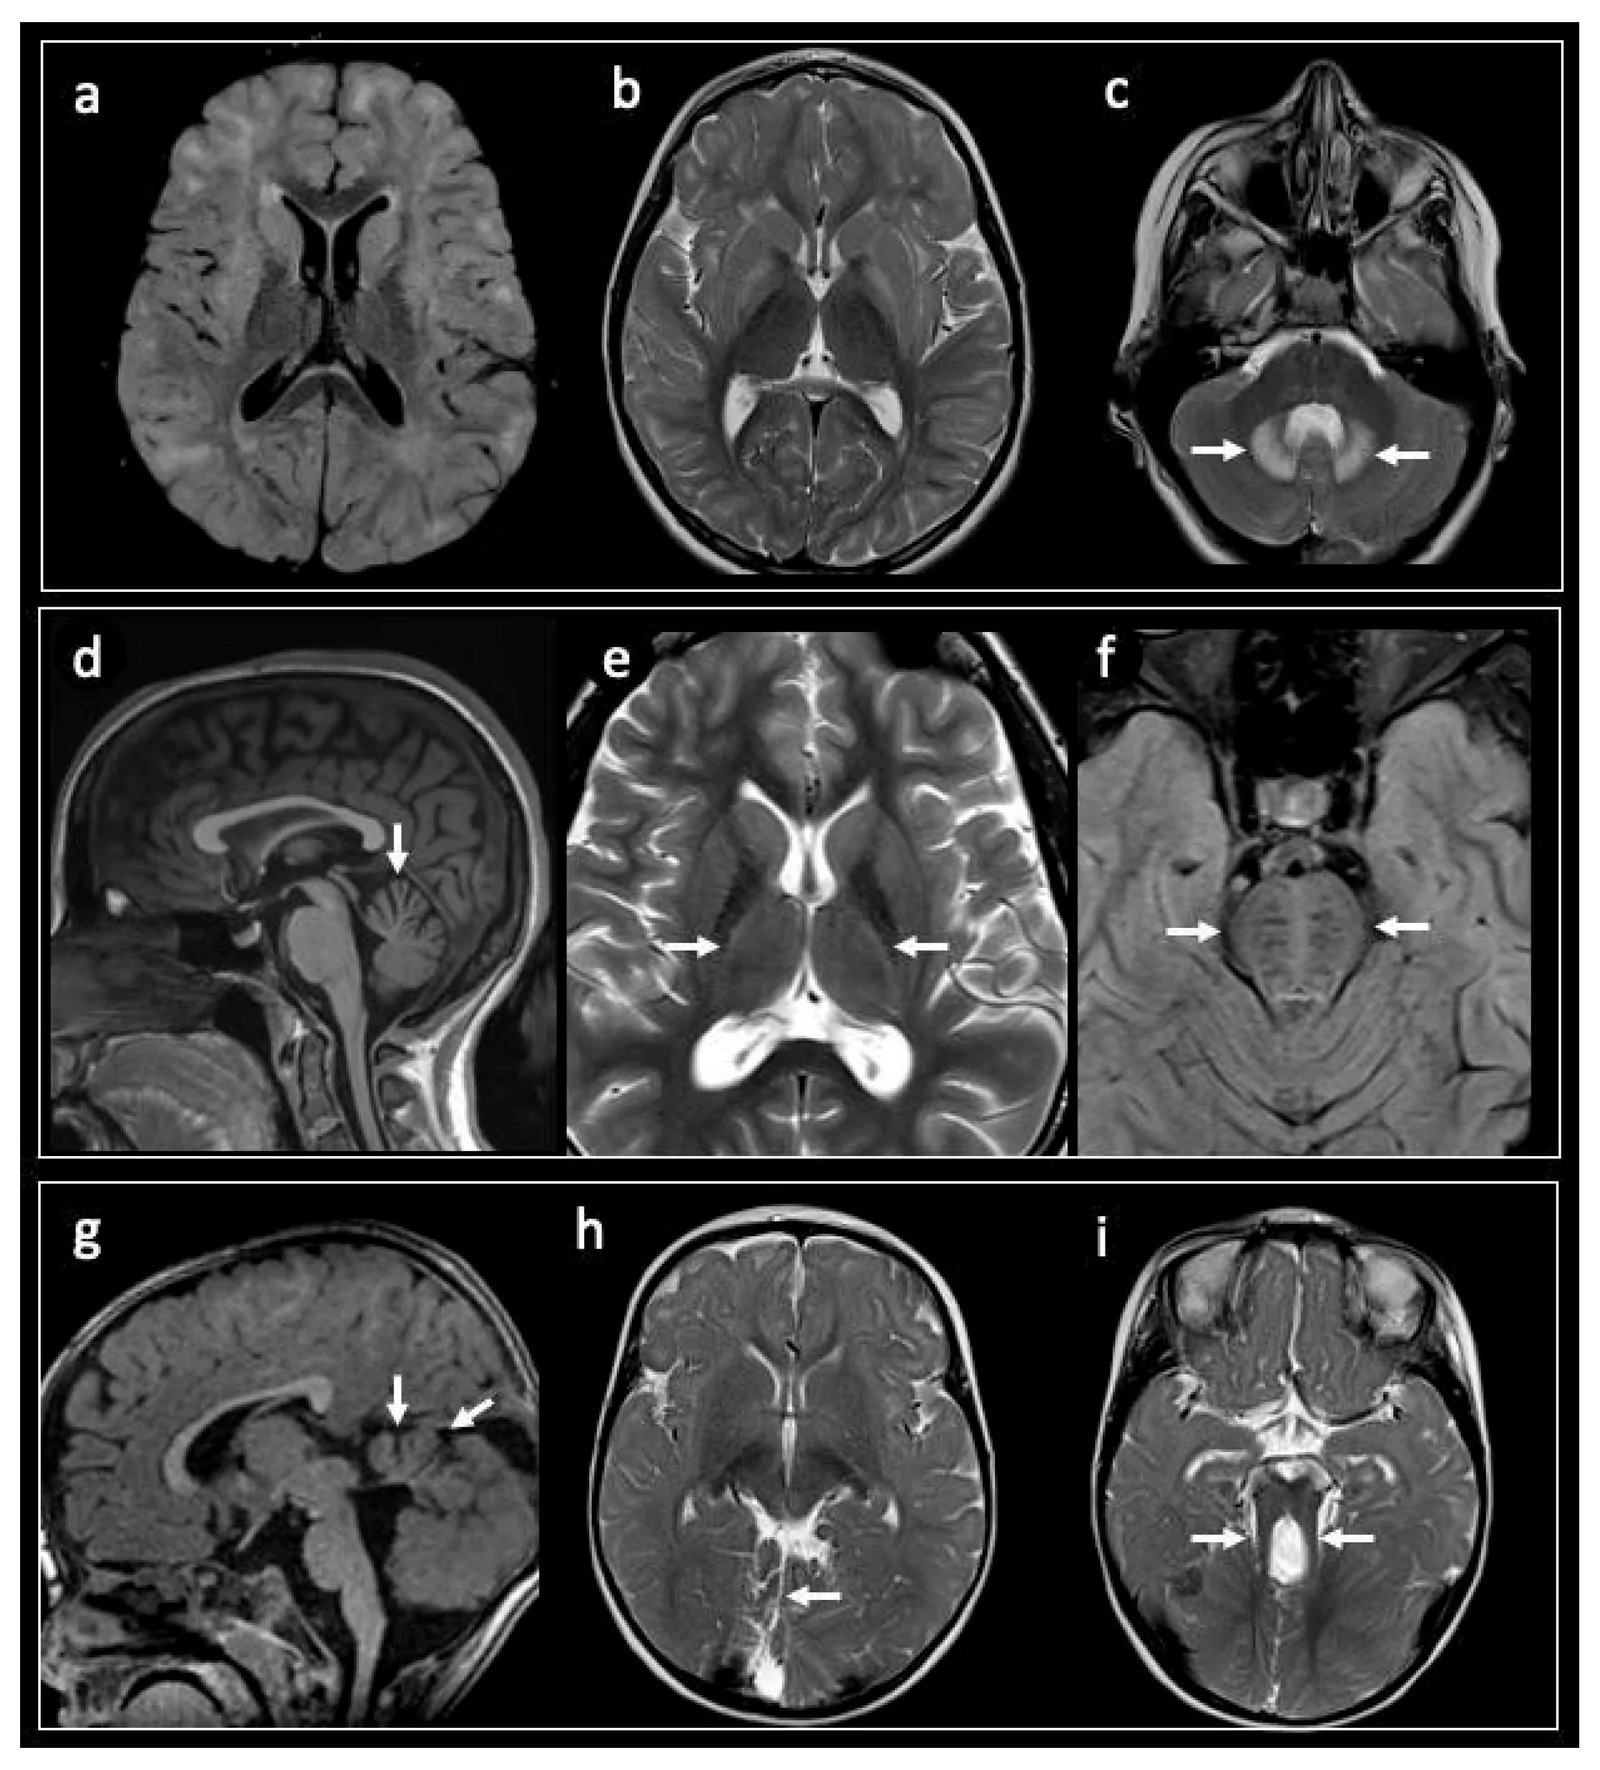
(a–c) L-2-hydroxyglutaric aciduria: Axial FLAIR images (a) show extensive white matter hyperintensities involving primarily subcortical white matter and U fibers. Axial T2-weighted images (b,c) also show involvement of the basal ganglia and dentate nuclei (arrows in (c)). (d–f) Autosomal recessive spastic ataxia of Charlevoix-Saguenay (ARSACS): Sagittal T1-weighted image (d) shows atrophy of the superior vermis (arrow) and inferior vermis part of the vermis is spared with relatively large pons. Axial T2 image (e) demonstrates T2 hyperintense rim around the thalami (arrows). Axial FLAIR image (f) shows the typical striated appearance of the pons (arrows). (g–i) Joubert syndrome: A sagittal T1-weighted image (g) shows small dysplastic vermis (arrows). Axial T2 images (h,i) demonstrate respectively median cleft of the vermis (arrow in (h)) and characteristic “molar tooth” sign with elongated superior cerebellar peduncles (arrows in (i)).
